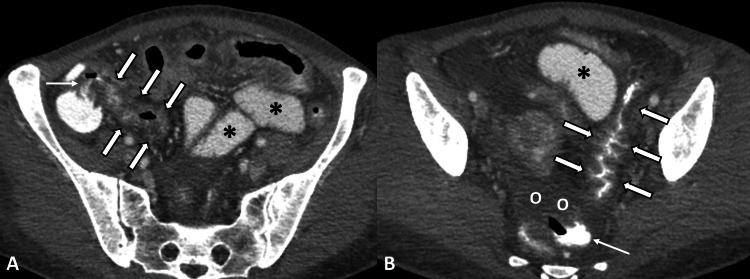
Abdominal CT scan in the portal venous phase (A) Transverse image at the level of the ileocecal valve shows mural thickening of the distal ileum with luminal narrowing (thick arrows), edematous appearance of the iliocecal valve (thin arrow) and marginally dilatated ileal loops (*). (B) Transverse image through the distal colon shows marked wall thickening of the proximal sigmoid colon with effacement of the lumen (thick arrows), dilatated ileal loops and free intraperitoneal fluid (O). Note contrast-filled rectum (thin arrow), indicating non-obstructive ileus.
